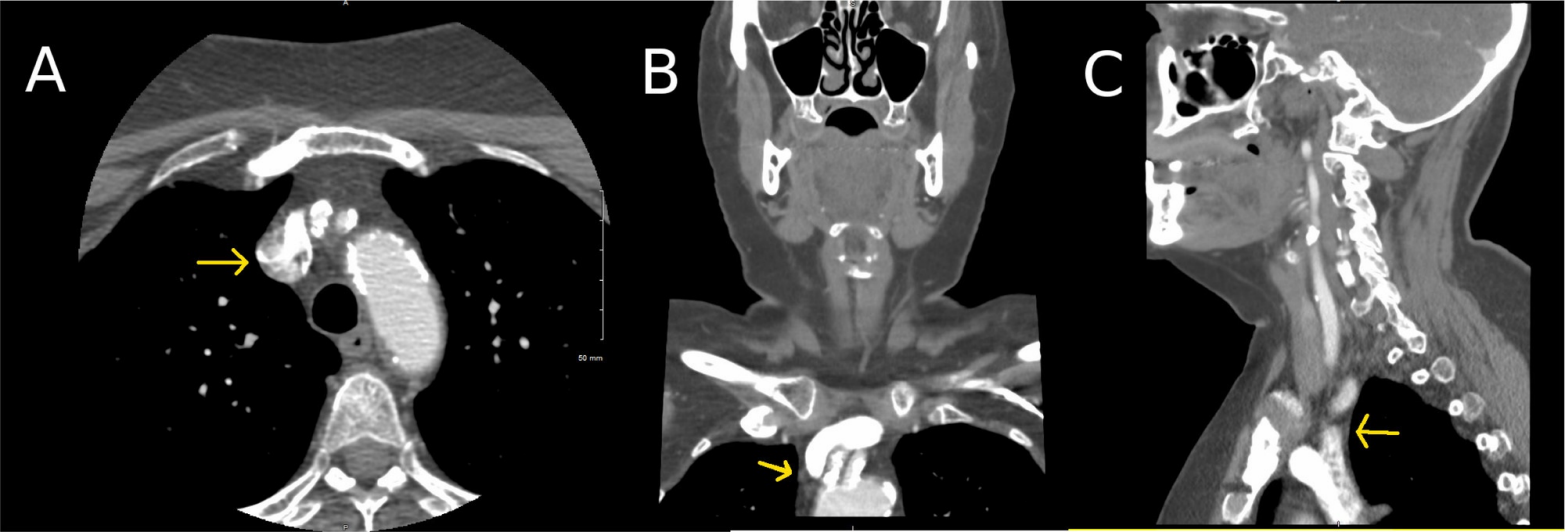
CTA of the neck showing 80% stenosis at the origin of the innominate artery (A) Axial View; (B) Coronal View; (C) Sagittal View CTA: Computed tomography angiography
